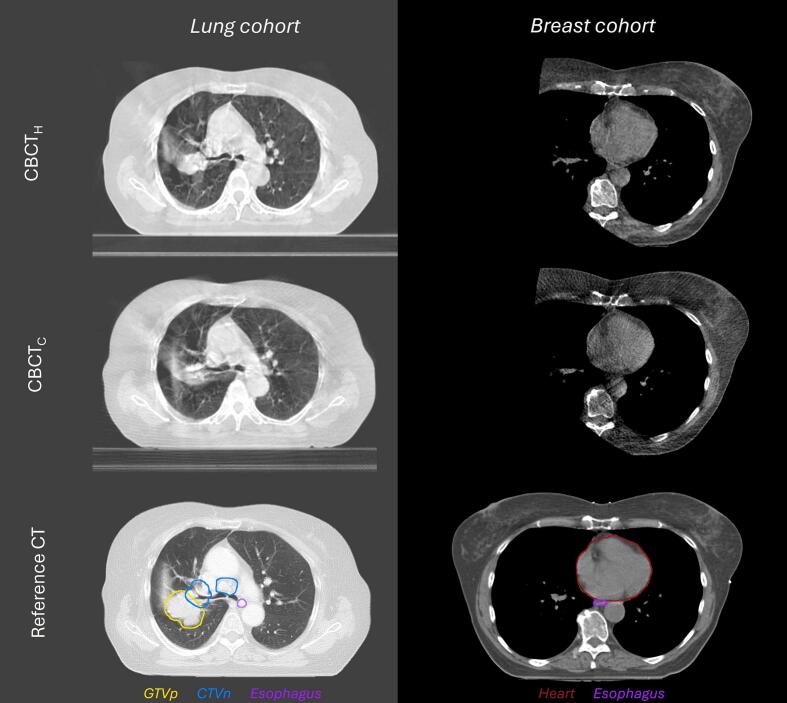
Examples of CBCT_H_ (first row), CBCT_C_ (second row) and reference CT with reference structures (third row) at corresponding axial slices for the lung cohort (first column) and breast cohort (second column).
